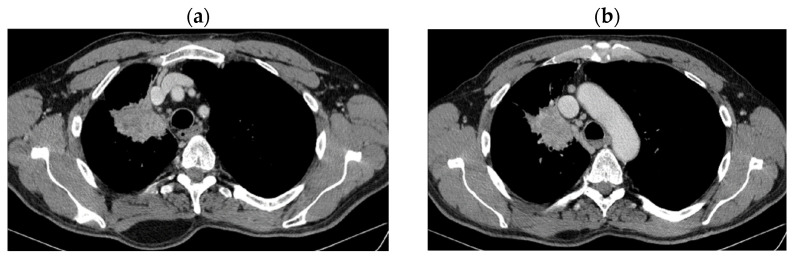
Progression of the disease: (a) Thoracic CT hilar nodes; (b) mediastinal nodes.
